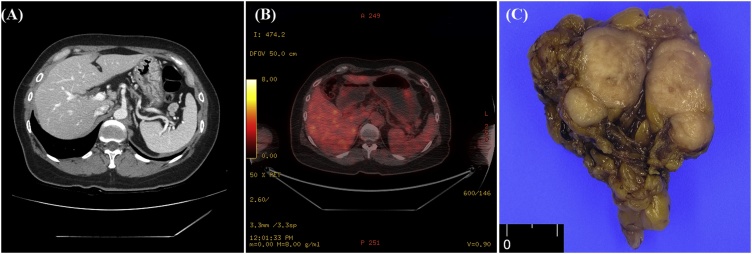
(A) Computed tomography findings of the abdominopelvis. Several enlarged lymph nodes are noted at the gastrosplenic area. (B) ^18^F-fluorodeoxyglucose positron emission tomography identified mild uptake (standardized uptake value max 2.8) in the gastrosplenic area. (C) Gross examination showed oval solid mass measuring 2.2 × 1.3 cm.
